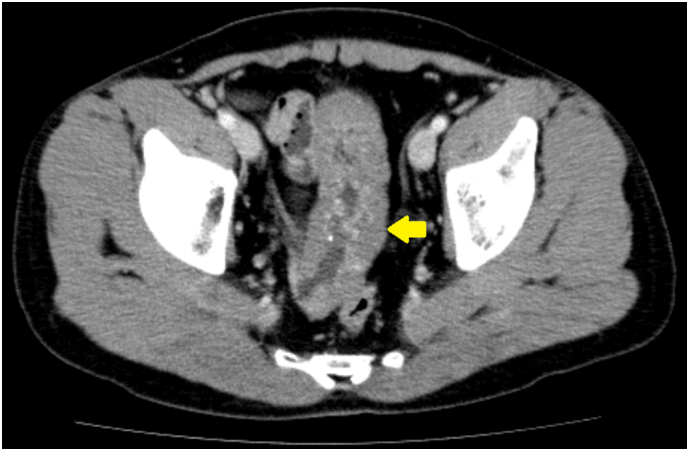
Preoperative computed tomography (CT) scan. Contrast-enhanced abdominal CT scan in a 29-year-old man who presented with chronic diarrhea, showed a 15 cm irregular thickening in the ileum, with focal calcifications.
